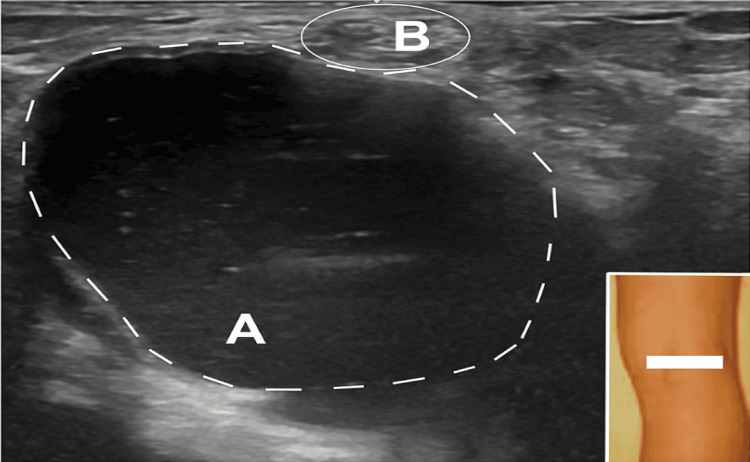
Ultrasound of the popliteal fossa Case #2: ultrasound (short axis view) at the popliteal fossa showing (A) Baker’s cyst (surrounded by white dashes) and (B) tibial nerve (encircled by an oval)
